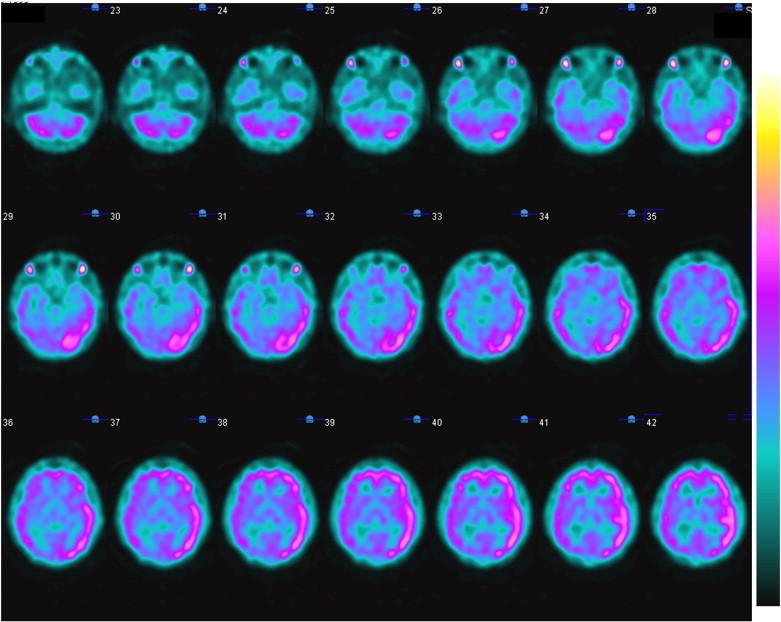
Perfusion SPECT imaging of the brain performed with Tc 99m EDC (Neurolite). 2D grayscale and color maps were produced using iterative reconstruction with and without attenuation correction. There is asymmetrically increased radiotracer uptake throughout the left cerebral hemisphere in comparison to the right, which is most pronounced in the left occipital and posterior parietal lobes. These findings correspond to the areas of signal abnormality and gyral swelling in the left cerebral hemisphere on MRI and suggest that images were acquired during seizure activity, likely an ictal SPECT.
